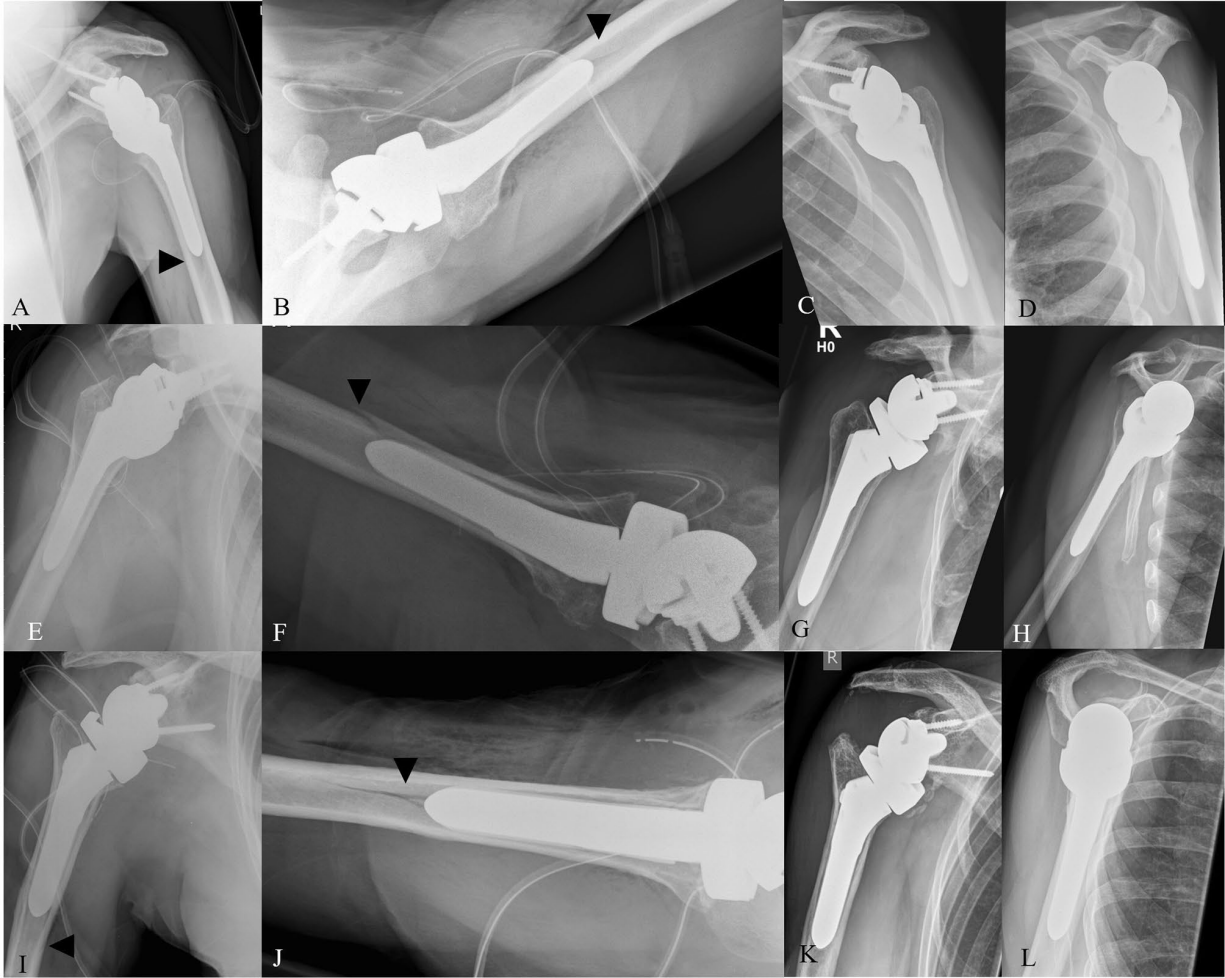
These radiographs show various fracture types with there follow-up. A/B 65-year-old man with irreparable rotator cuff tear. On the initial radiograph after the implantation of a RTSA, a non-displaced, oblique humeral shaft fracture is visible at the distal end of the stem on the anteroposterior as well as on the axial view. C/D 5-year follow-up of the case. No radiolucency of the shaft is visible. E/F Radiographs of a 72-year-old woman treated with a RTSA for irreparable rotator cuff lesion. The postoperative radiographs show a slightly displaced, spiral humeral shaft fracture. The fracture is only visible on the axial view. G/H 2-year follow-up. No radiograph complication can be detected at last follow-up. I/J Radiographs of a 79-year-old woman, treated with a RTSA because of irreparable rotator cuff tear. Also, in this case, the hypodense fracture line is only visible on the axial view and seems to be bifurcated. K/L 5-year follow-up of the case. No radiolucency of the shaft is visible.
